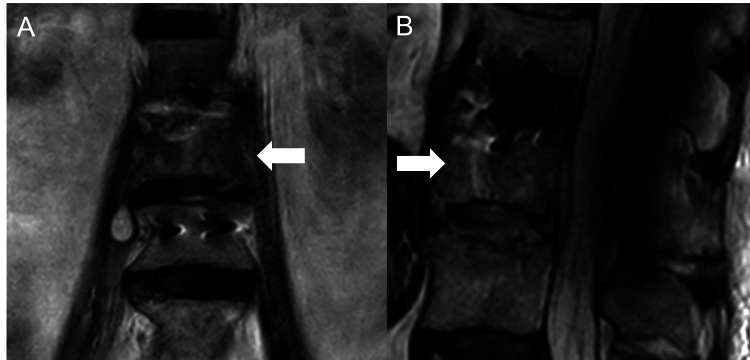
Postoperative magnetic resonance imaging of the lumbar spine (A) coronal image, (B) sagittal image T2-weighted magnetic resonance imaging showed a reduction in the iliopsoas and epidural abscesses. Arrows: L3 vertebral body
